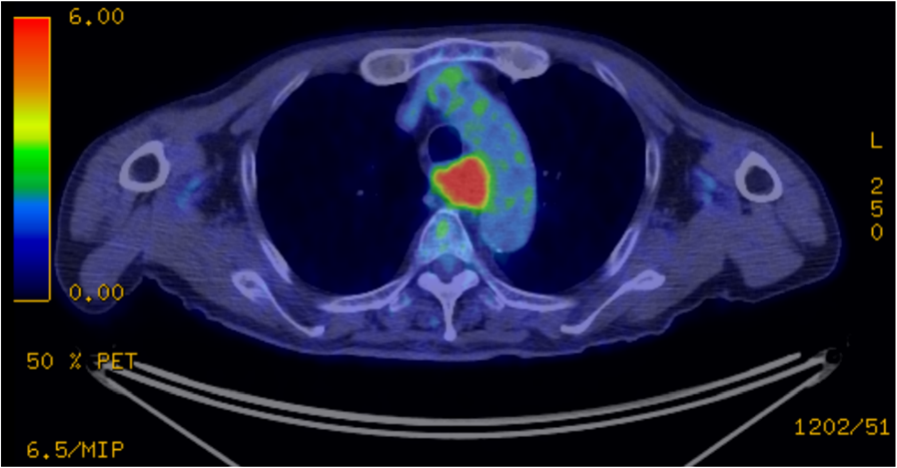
Positron emission tomography–computed tomography: There is radionuclide accumulation in the portion where the *en bloc* mass forms an esophageal tumor compressing the membranous portion of the trachea and lymph node metastasis is present, but there is no evidence of distant metastasis
